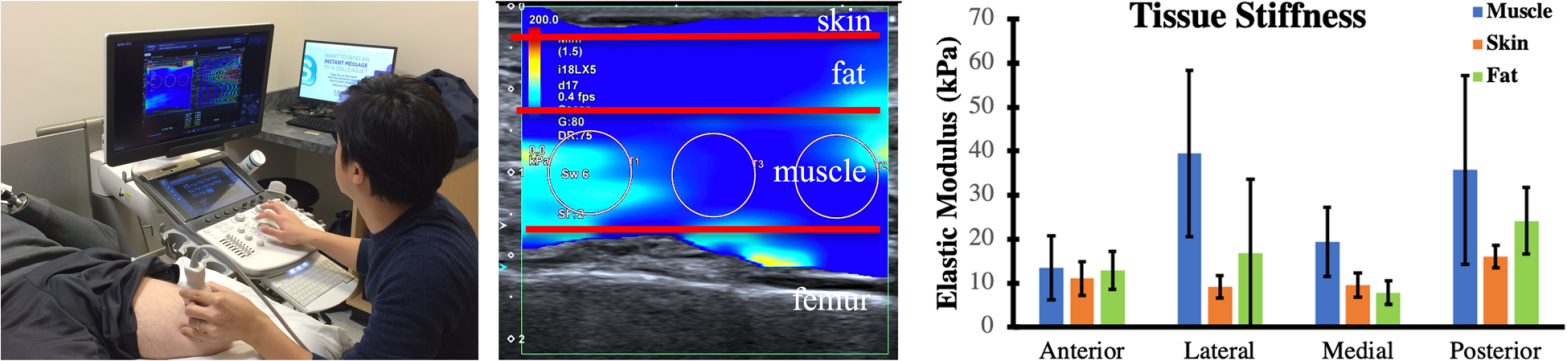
Ultrasound elastography performed on the residual limb of a transfemoral amputee (left). Tissue stiffness is color mapped onto the original ultrasound image and measured within 3 regions of interest (middle). Average values within each region are determined for each tissue (right)
